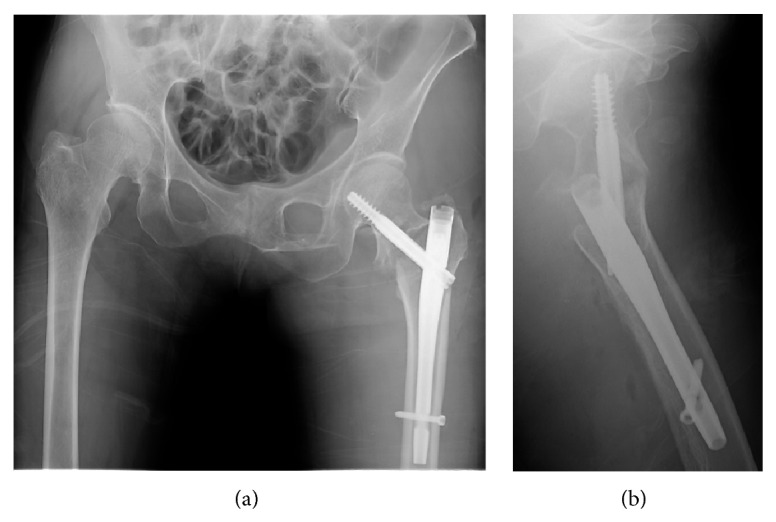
Radiographs after the osteosynthesis of the left trochanteric fracture. Anteroposterior (a) and lateral (b) views of the left femur showing insufficient reduction of the trochanteric fracture after implantation of a gamma 3 nail.
